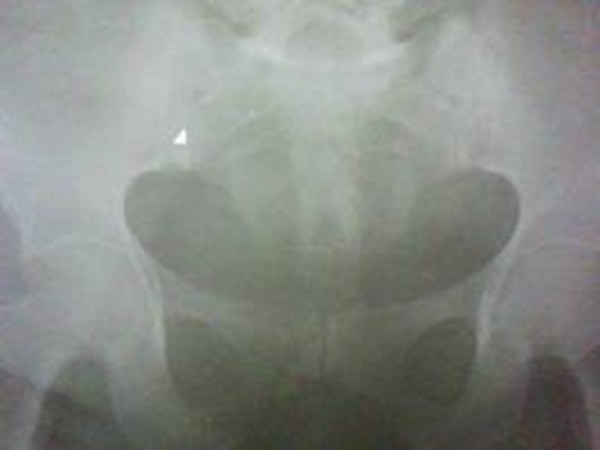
Anteroposterior radiographs of the pelvis showing asymmetric erosive sacroileitis on the right side.
